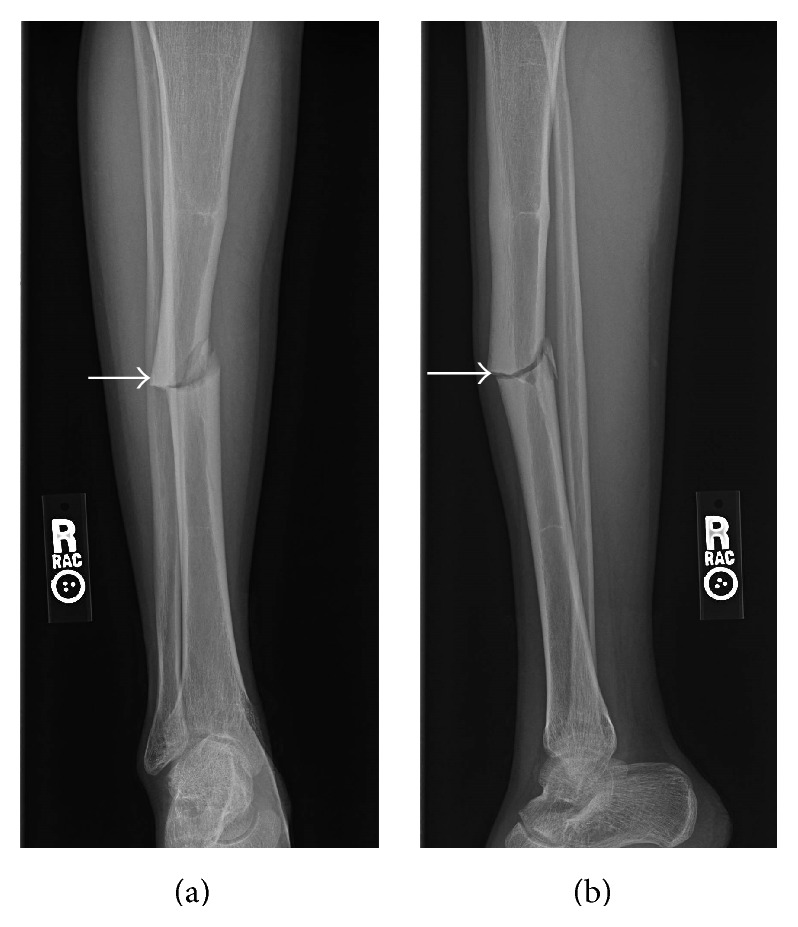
Young adult male with history of Rothmund-Thomson syndrome diagnosed at 2 years of age. Findings: (a) mildly comminuted and mildly displaced fracture through the mid diaphysis of the right tibia. There is lateral cortical thickening at the level of the fracture (arrow). (b) Mildly displaced fracture through the mid tibia diaphysis with focal anterior cortical thickening at the level of the fracture (arrow). Technique: (a) AP radiograph kVp = 66, mAs = 2, and (b) lateral radiograph kVp = 64, mAs = 2.
